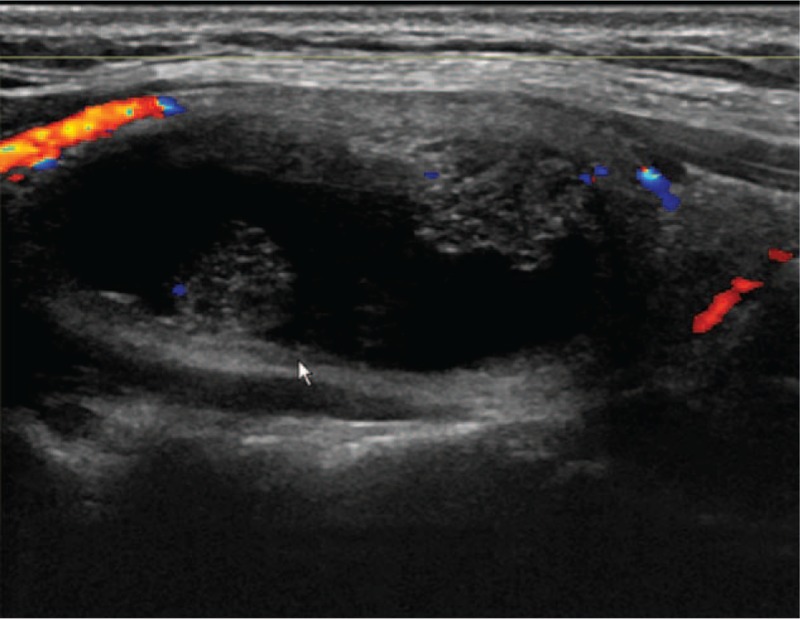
Longitudinal section of the nodule showed by pre-MWA ultrasound. MWA = microwave ablation.
